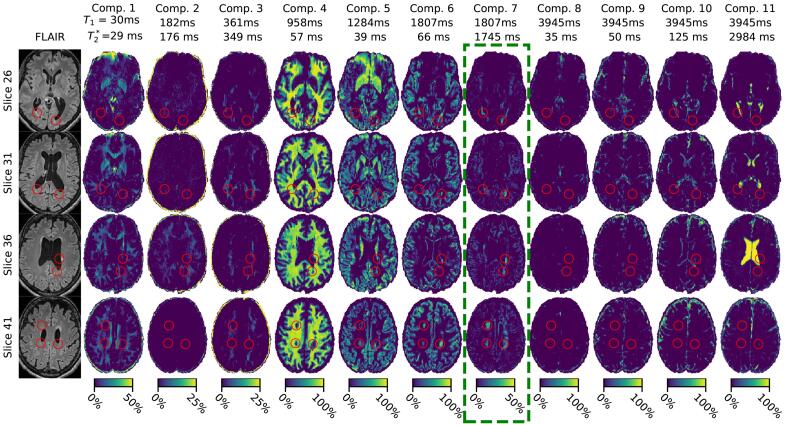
FLAIR images and obtained MC-MRF component maps a for a selection of slices of a representative MS patient. Estimated relaxation times are shown above each column. Note that color ranges differ per component for visualization purposes. Components are ordered by *T*_1_ relaxation time. Shown images are registered to correct for susceptibility distortion. The green box indicates the component of interest. The red circles indicate white matter damage as visible in component 7. Component 2 shows a sharp increase in signal in slice 36 compared to the other slices. This is most likely caused by the different timing of RF-pulses per slice used in the MRF-EPI sequence. (For interpretation of the references to color in this figure legend, the reader is referred to the web version of this article.)
